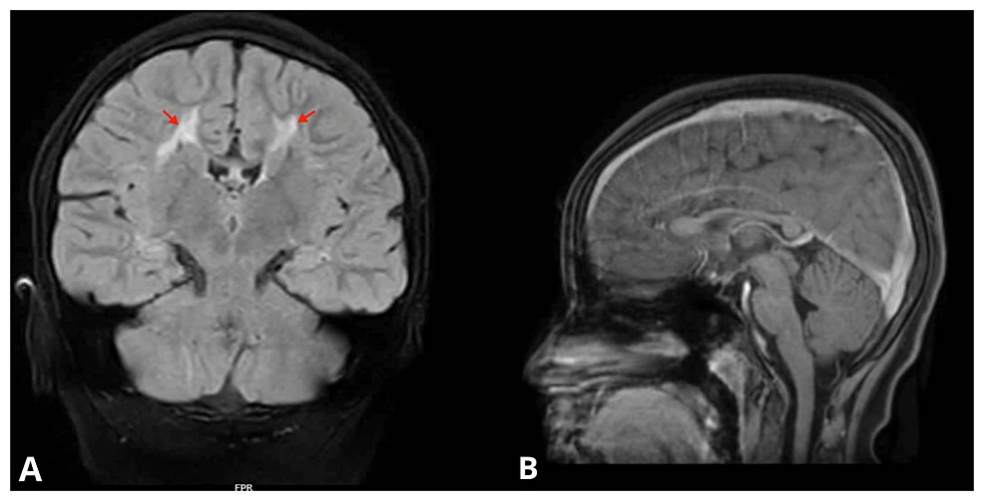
Symmetric gliotic signal with T2-FLAIR hyperintense signal (A) (coronal view) and T1 hypointense signal (B) (sagittal view). A: T2-weighted FLAIR, sequence 10. B: Sequence 15, T1-weighted. FLAIR: fluid-attenuated inversion recovery
